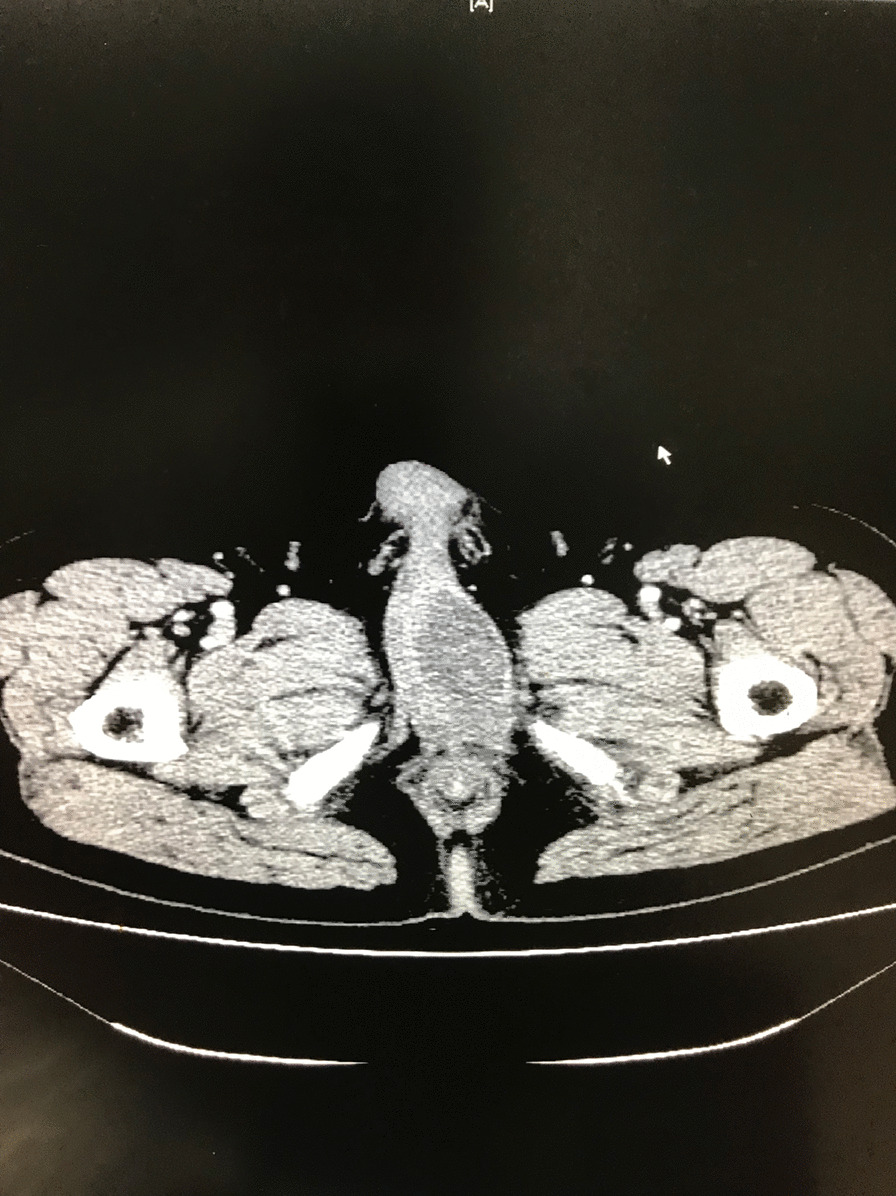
Computed tomography imaging. A well-defined, irregular, and heterogeneously enhancing soft tissue mass arising from the proximal part of the penis
